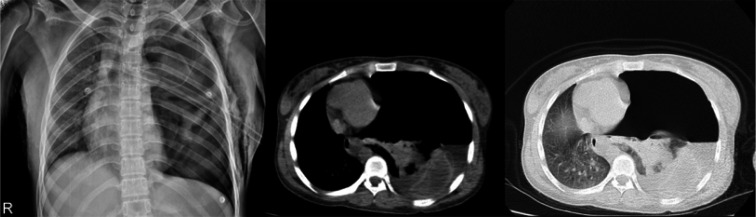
Posterior-anterior chest X-ray (left) and axial computed tomography (CT) scan in the mediastinal and lung window (middle and right) of patient
